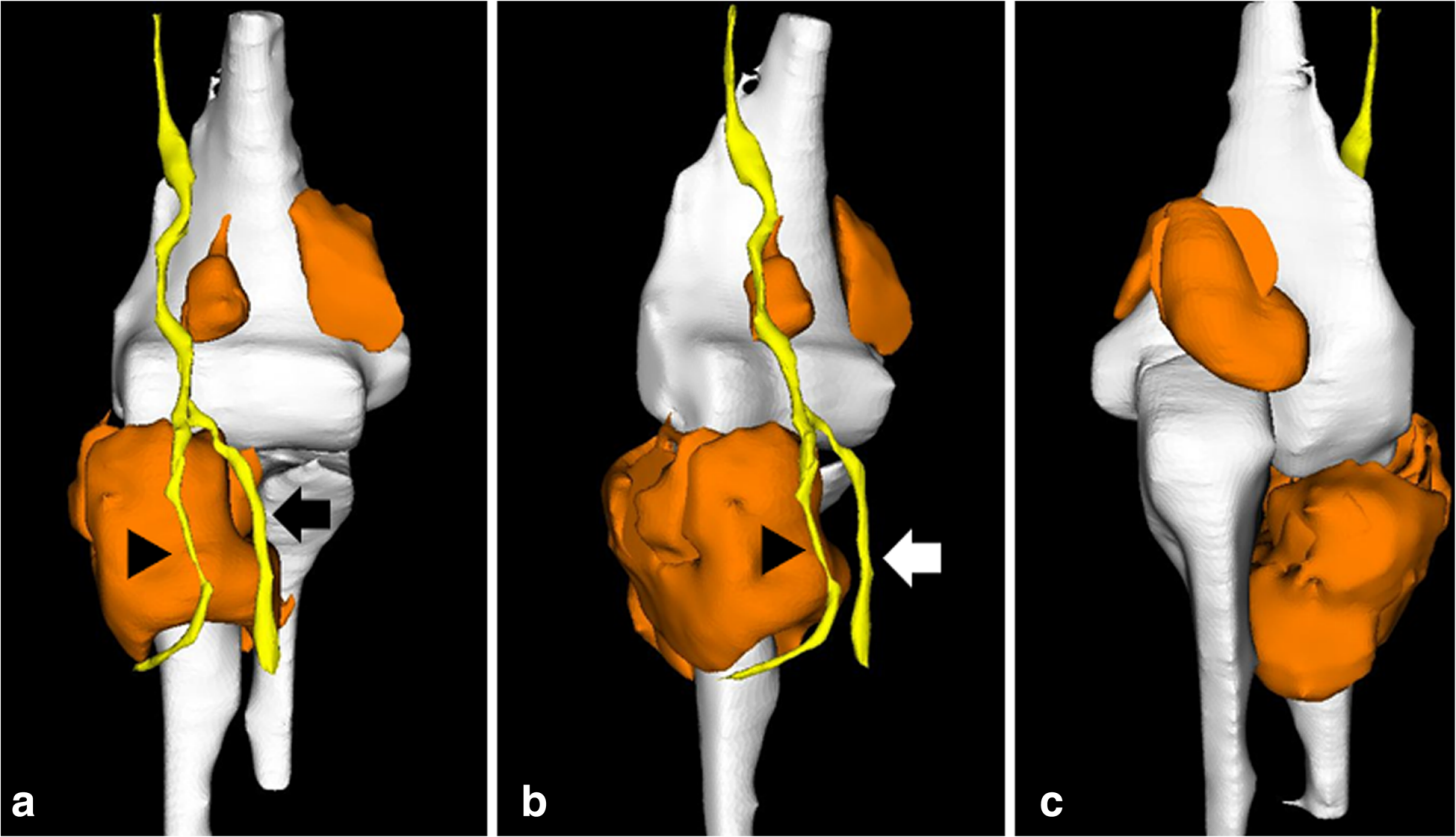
Three-dimensional images based on magnetic resonance imaging of the right elbow. a Anterior image. b Oblique image. c Posterior image. The tumor is orange; the bone, humerus, radius, and ulna are white; and the nerve is yellow. The running course of the posterior interosseous nerve (*arrow head*) is changed at the distal corner of the tumor in contrast to the superficial branch of radial nerve (*arrow*)
